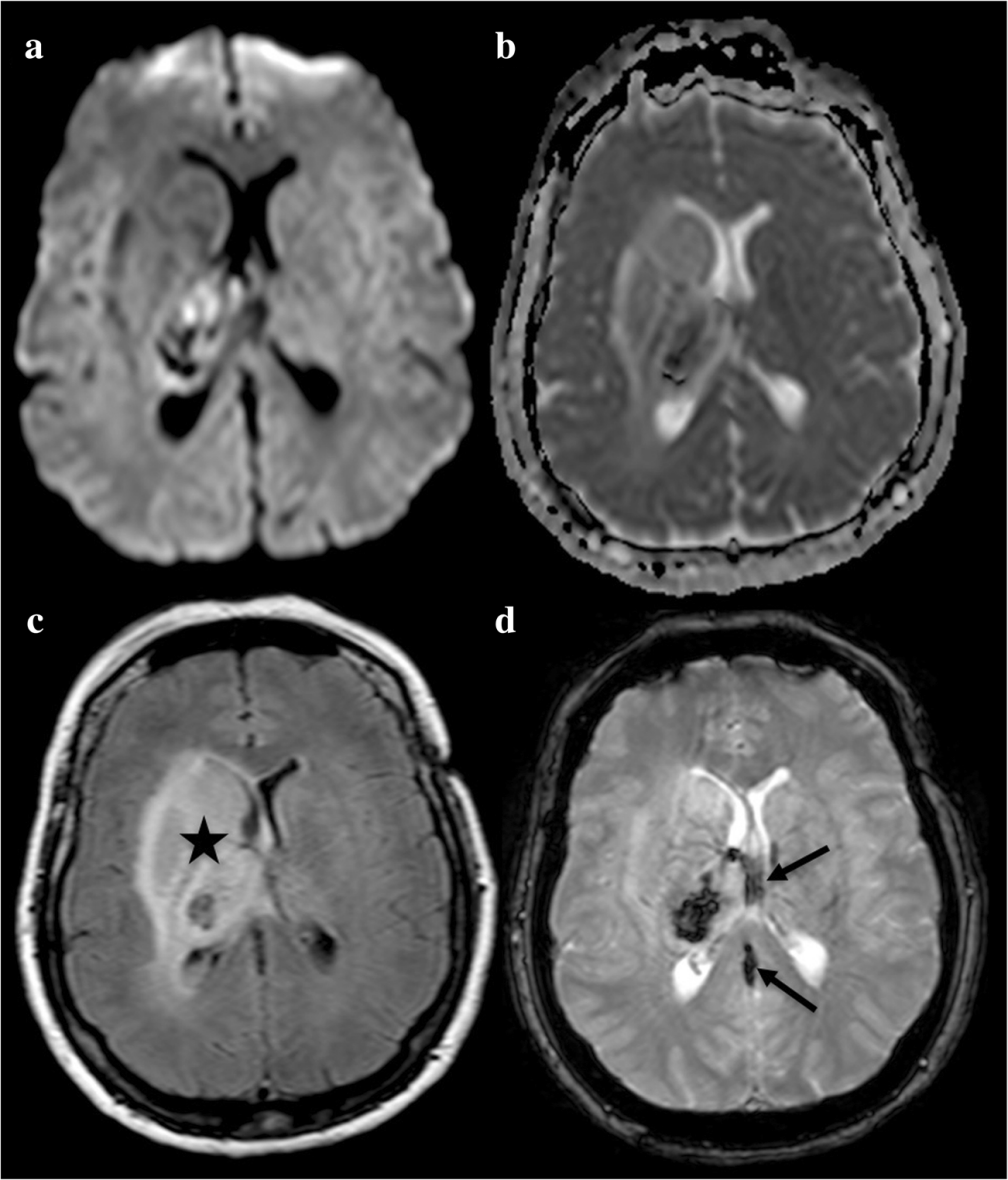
A 50-year-old woman with cerebral venous thrombosis presenting with drowsiness and left hemiplegia. DWI shows heterogeneous signal abnormalities on b1000 (a) and ADC (b). Hypersignal FLAIR swelling (c, star). T2-GRE shows an artefactual hypointensity in the right internal cerebral vein, the great vein of Galen and the straight sinus (d, arrows)
